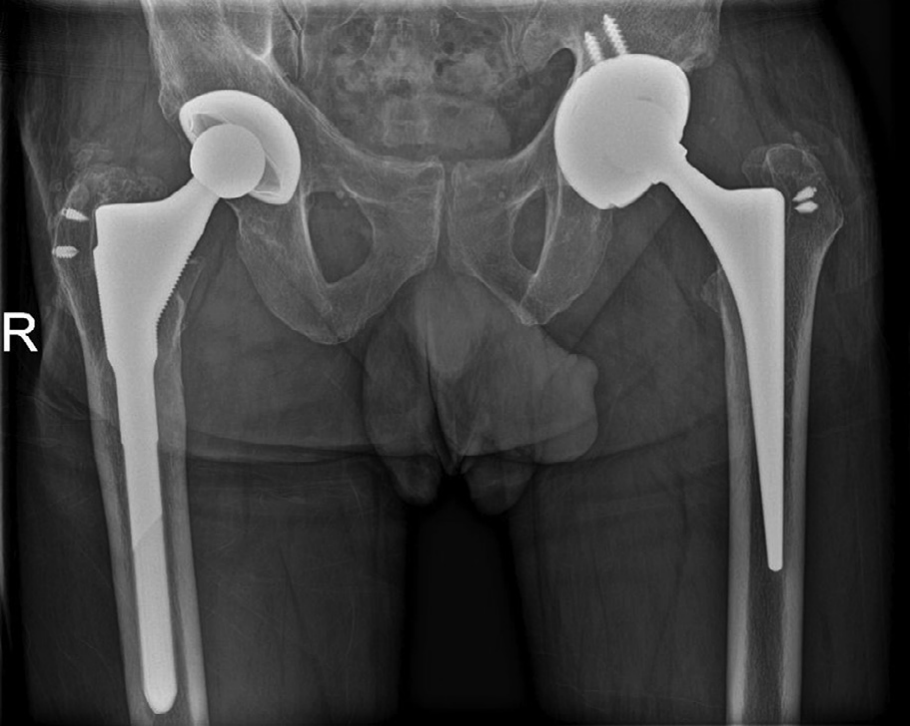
Post-operative low-centered AP radiograph of the pelvis demonstrates interval revision of the left total hip arthroplasty, now with a metal-on-polyethylene design. The right hip arthroplasty is unchanged.
